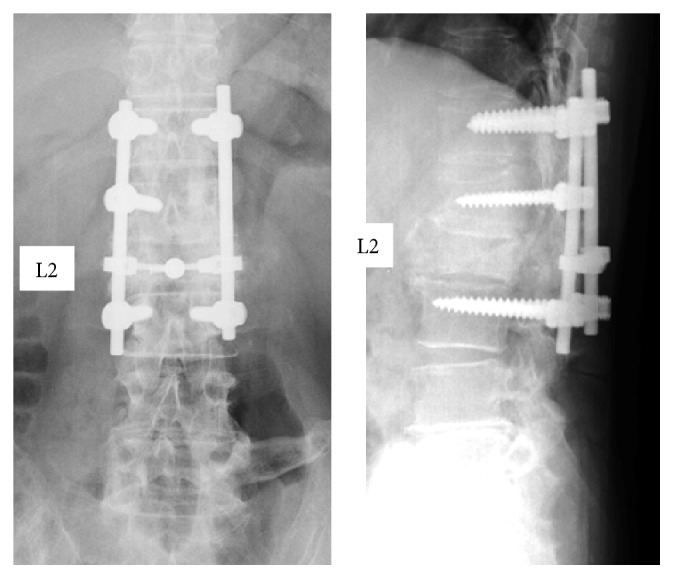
X-ray image of the spine at the first visit. Deformation of L2 vertebral body after posterior fixation surgery.
